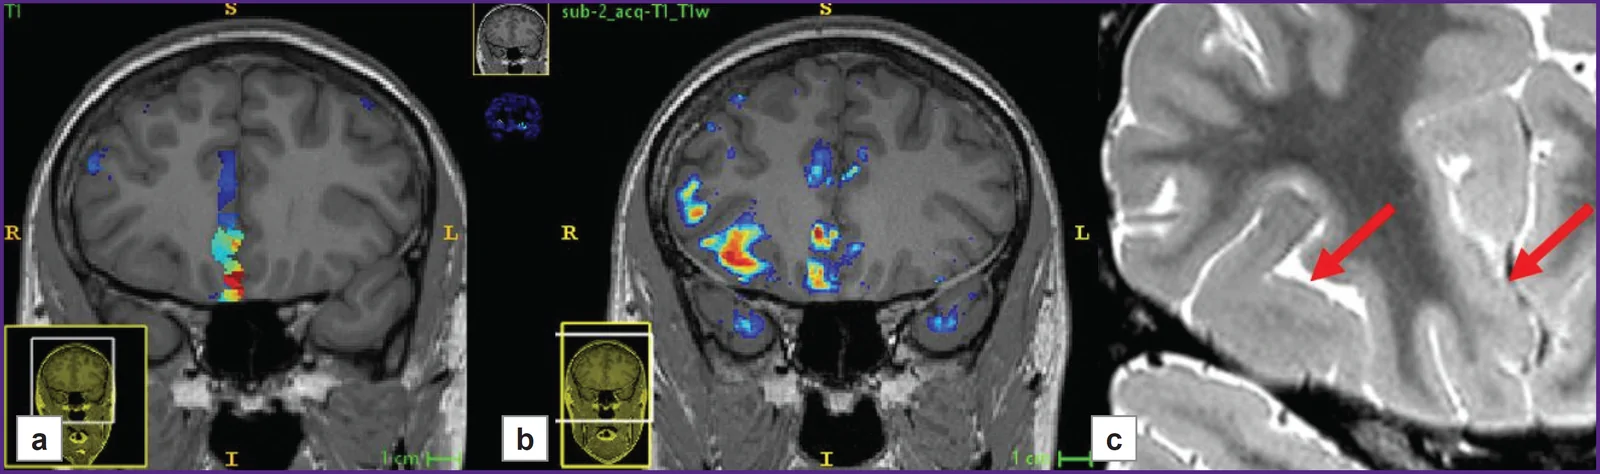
MRI of a 21-year-old woman with multifocal focal cortical dysplasia in the right frontal lobe: (a) classical morphometry: a lesion identified only in the straight gyrus; (b) multimodal morphometry: two affected areas detected (straight and inferior frontal gyri); (c) T2-weighted MRI revealing two zones of focal cortical dysplasia (straight and inferior frontal gyri), with the arrows marking the areas of alteration
